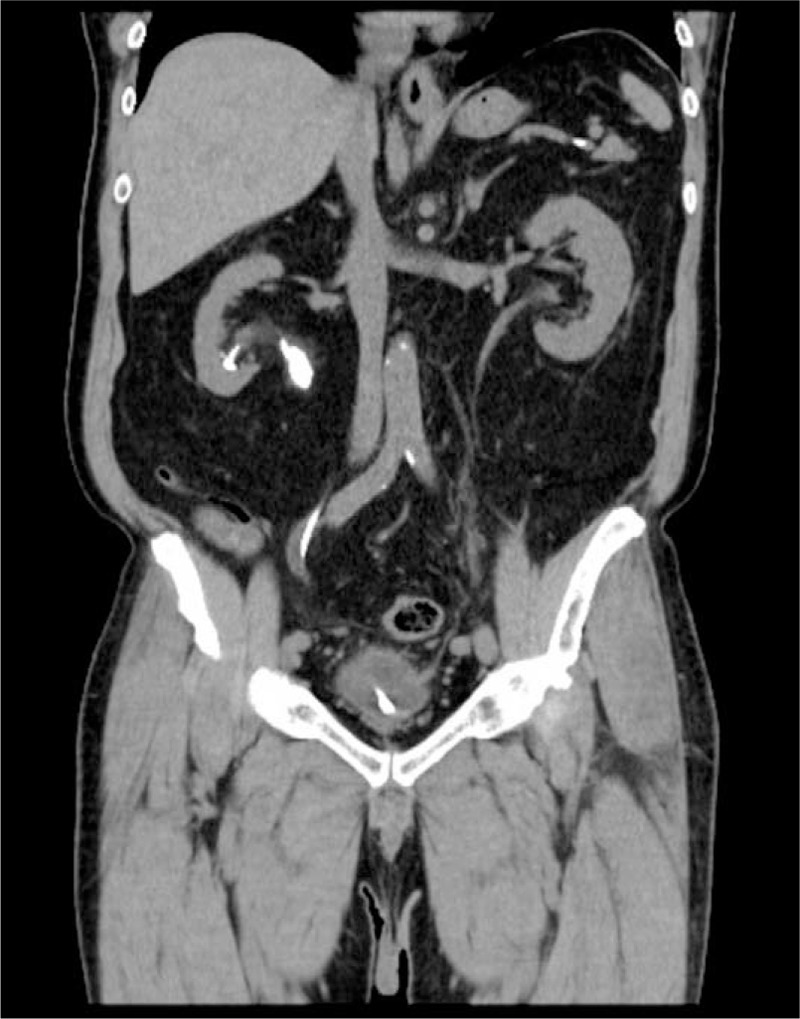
Preoperative computed tomography was arranged to identify the relative positions of the ureteropelvic junction stone, renal stones, and D-J stent. D-J = double-J catheter.
